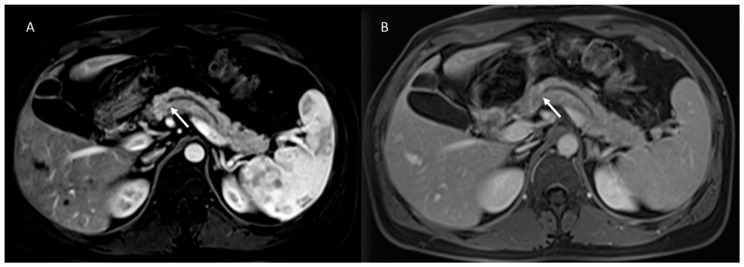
The same patient as in Figure 2; post-contrast MRI evaluation. In arterial (A) and portal (B) phase of contrast study, arrows show no tissue but dilatation of the main and secondary branch ducts.
